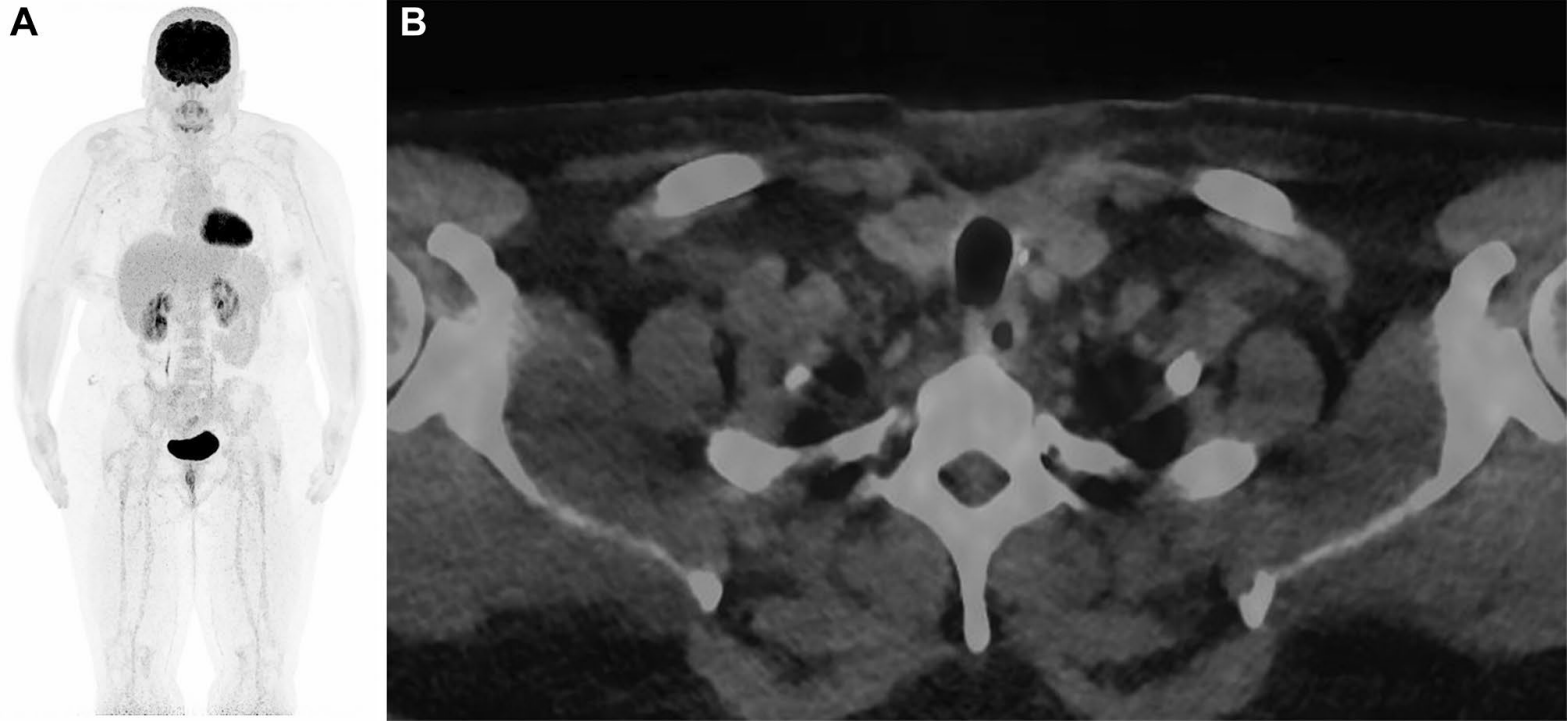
a and b FDG-PET/CT imaging 6 months postoperatively showing no sign of systemic or locoregional disease
